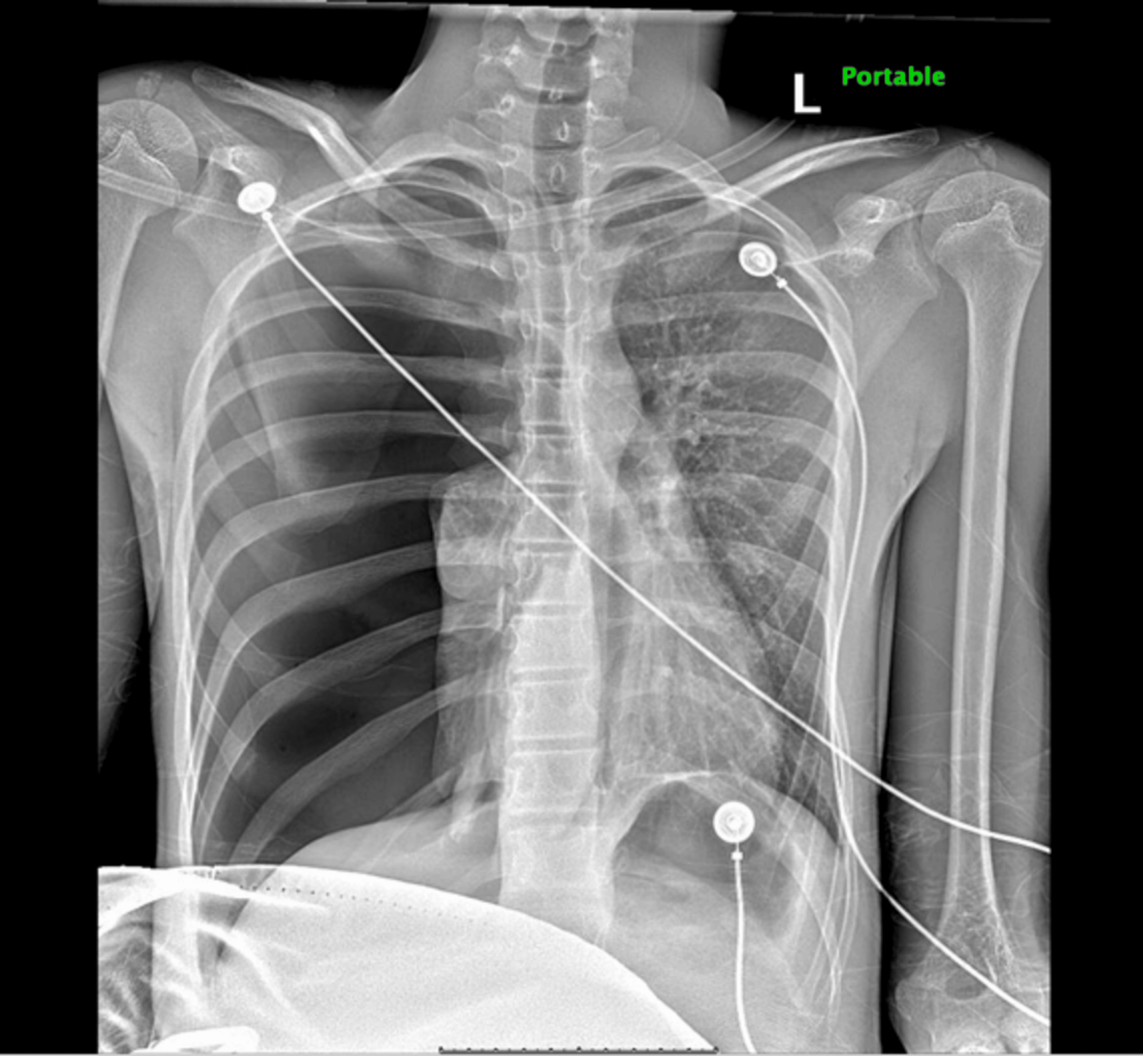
Chest X-ray demonstrating right-sided pneumothorax with associated right lung collapse
